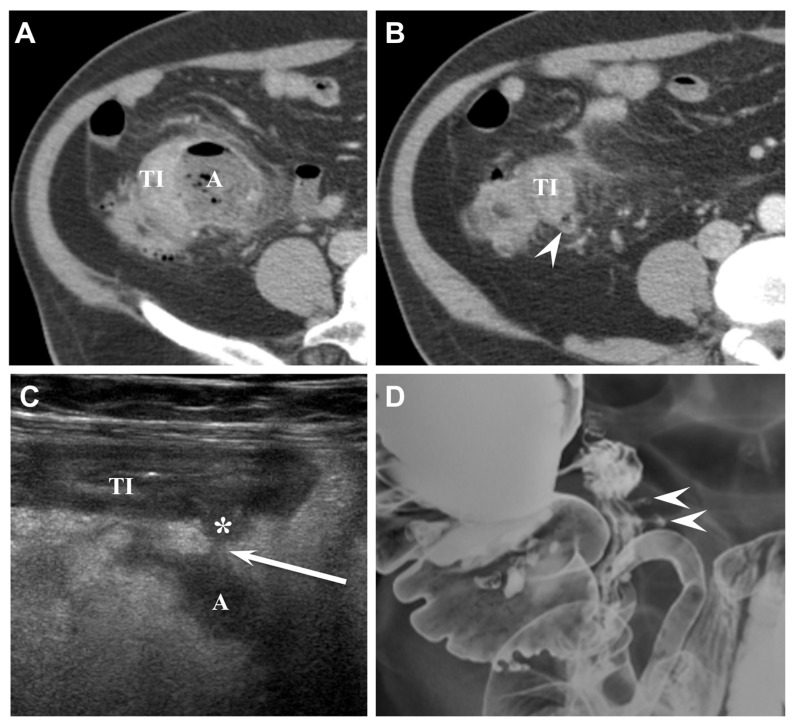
Sixty-seven-year-old male (patient #2) with acute perforated ileal diverticulitis. (A,B) Axial CT scans show ileal wall thickening (TI) with mesenteric abscess (A) and mesenteric fat infiltration. There is no definite inflamed diverticulum on CT. However, adjacent ileal diverticulum (arrowhead) supports the diagnosis of perforated ileal diverticulitis with abscess formation. (C) US scan with linear transducer reveals outpouching inflamed diverticulum (*) protruding from the terminal ileum (TI) and connecting to mesenteric abscess (A) with focal perforation site (arrow). (D) Barium study after 1 month shows two diverticula (arrowheads) protruding from the terminal ileum and multiple diverticula from the colon.
